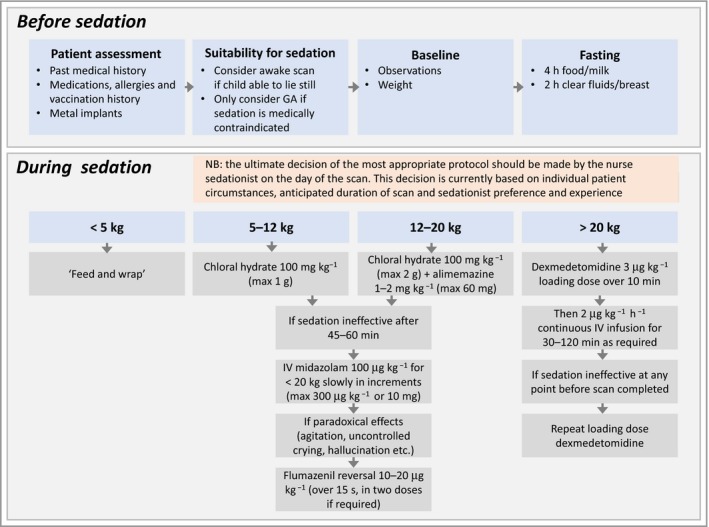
Current sedation protocol for paediatric magnetic resonance imaging used at Great Ormond Street Hospital for Children NHS Foundation Trust, London, and for which a 90% success rate was obtained in this cohort. Sedation is also cheaper than general anaesthesia (GA). IV, intravenous.
